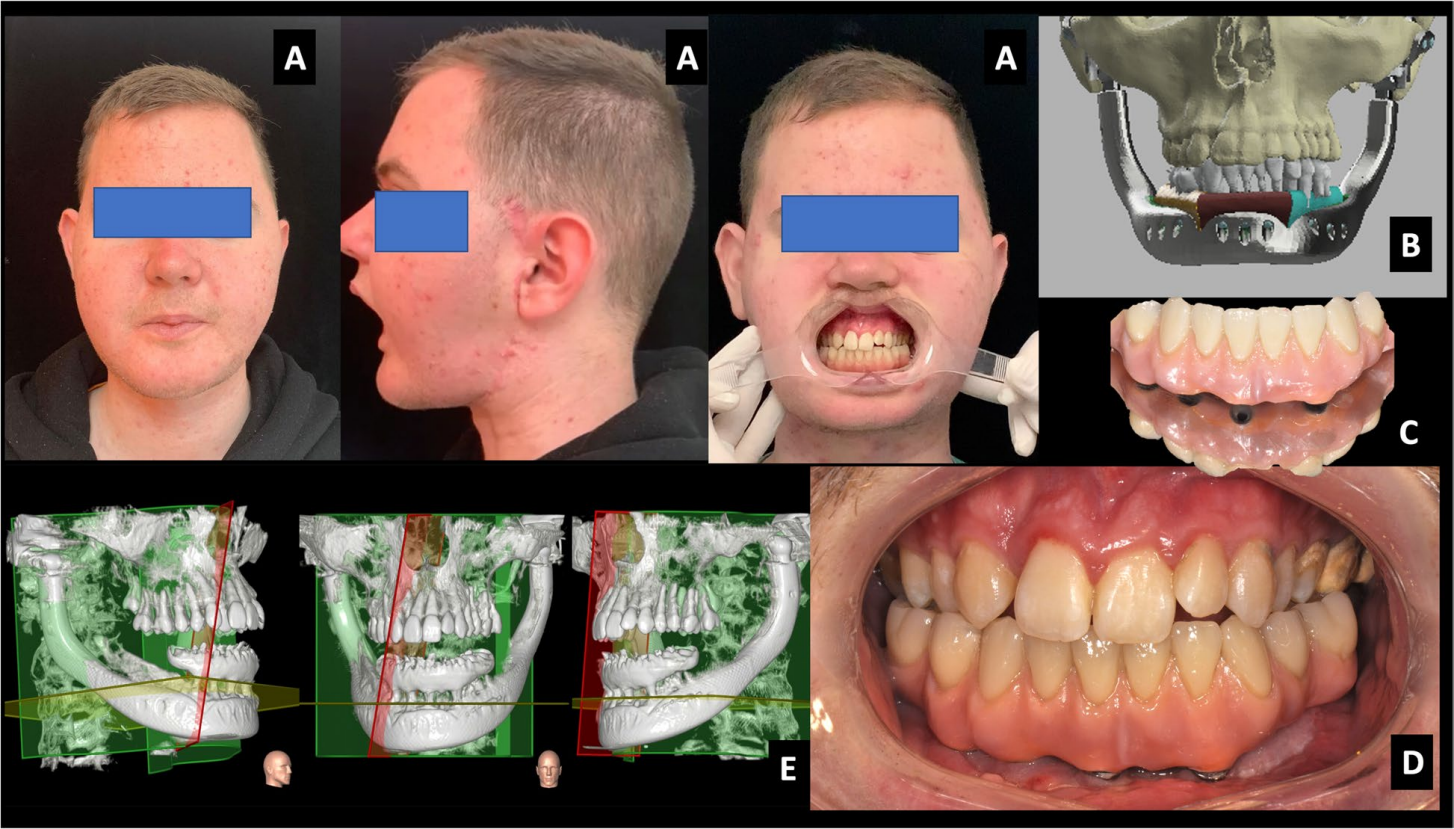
The final result at the 18^th^-month follow-up a patient views, note the mouth opening in lateral view and the dental occlusion, b the pre-treatment 3D-VSP (virtual surgical plan) in comparison with the obtained results, c the final dental prosthesis, d the 3D CT scan at the end of the treatment, and e the final occlusal view
